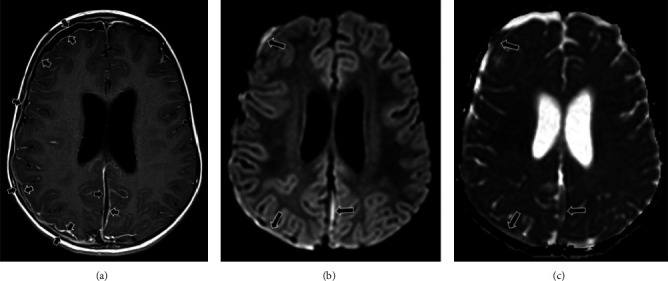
MRI day 1. Axial postcontrast T1 TSE SPACE (a), b1000 diffusion-weighted image (b), and ADC map (c). There is a right-sided and an interhemispheric subdural empyema, characterized by pathological pachymeningeal and leptomeningeal contrast enhancement (short arrows), and increased diffusion restriction (long arrows). Also, note the mild hydrocephalus.
